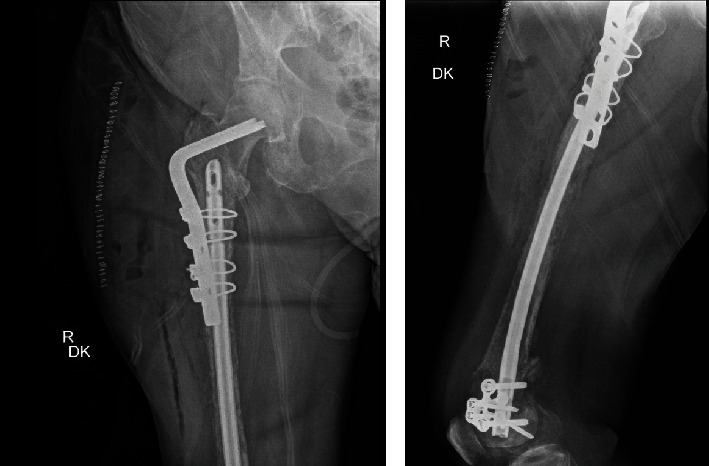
Postoperative radiographs of femur demonstrating fracture fixation with 95-degree angled blade plate and cerclage wires.
